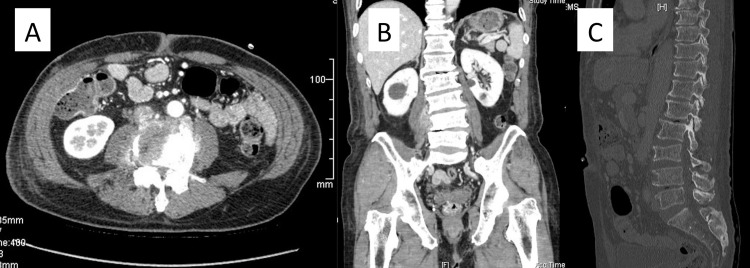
Enhanced computed tomography scan slices. (A) Axial slice shows an enhanced lesion around the L4 vertebral body and right psoas muscle, suggesting the focus of infection. (B) Coronal slice shows an enhanced lesion in the right psoas muscle, suggesting psoas abscess. (C) Sagittal bone quality slice shows no destructive changes in the L3/4 endplates and vertebral bodies.
